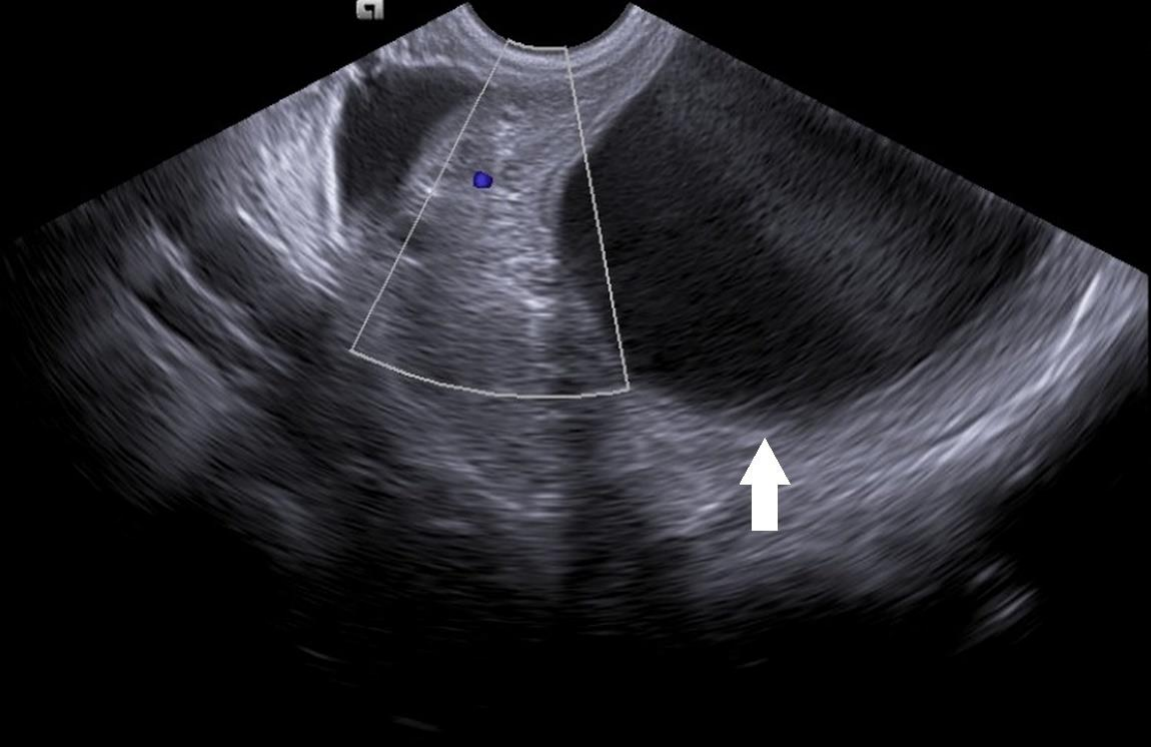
Transvaginal pelvic ultrasound showing right ovary with reduced vascularization on a Doppler study and the paraovarian cyst (white arrow).
